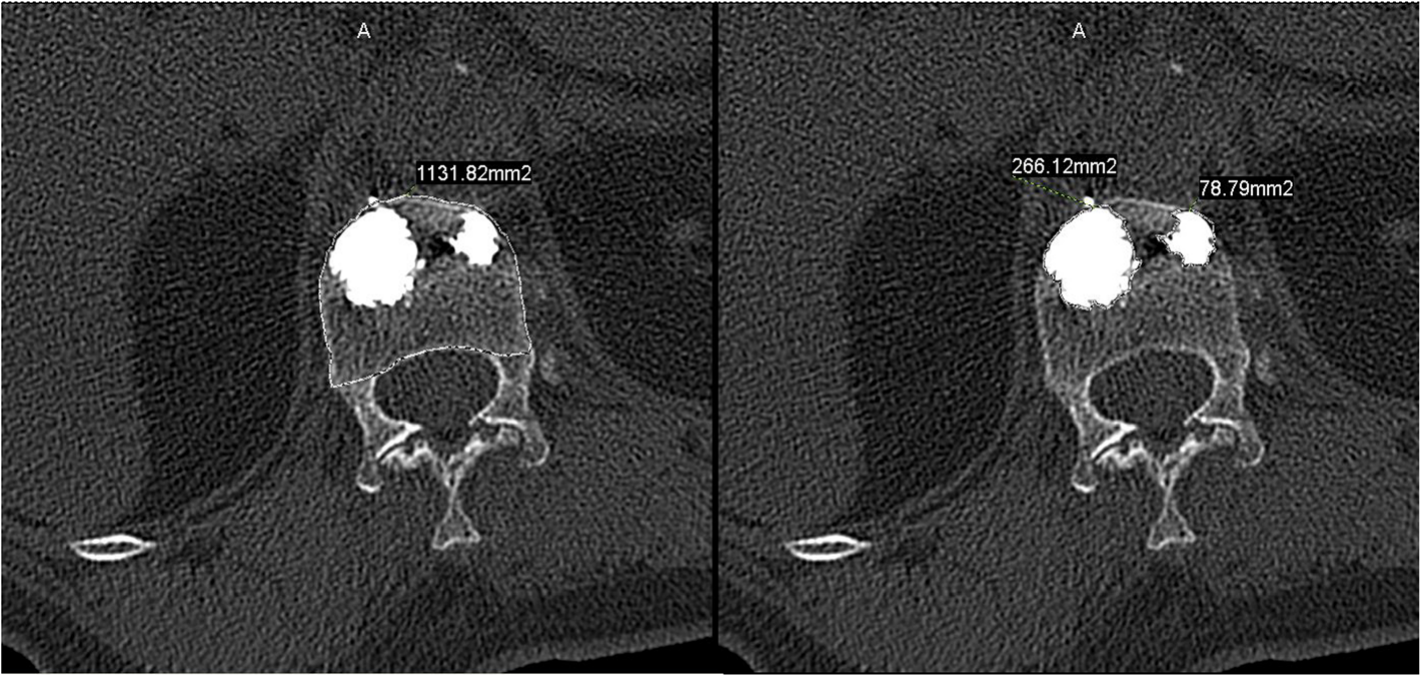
The cement border and the vertebral border in the axial CT images were manually outlined using the “Area measurement” tool in the PACS software, and the areas were automatically calculated
